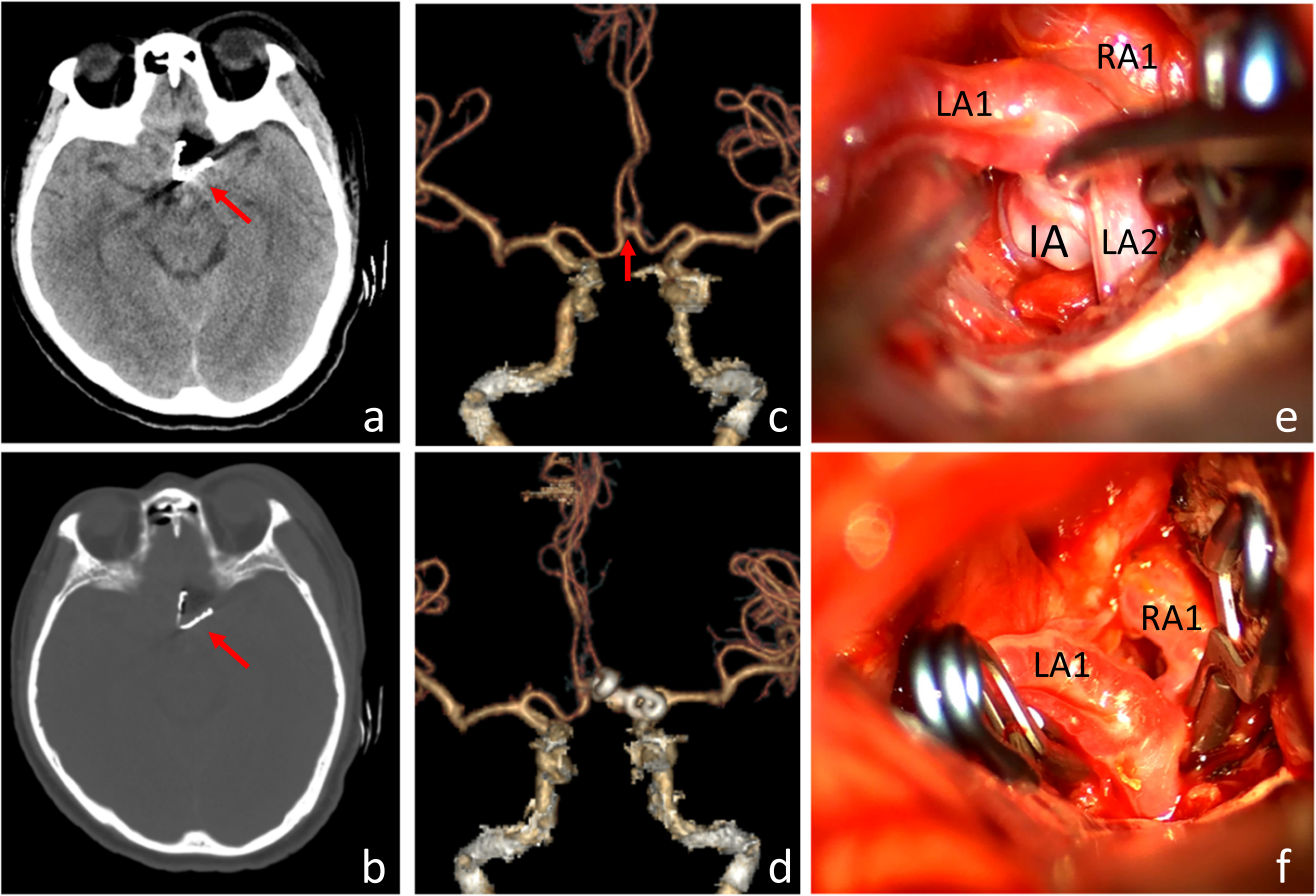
Preoperative and postoperative imaging and intraoperative findings of ruptured anterior communicating aneurysms with reconstruction clipping via supraorbital lateral keyhole approach. a, b The ruptured aneurysm of the patient's anterior communicating artery was shaped and clipped with 2 aneurysm clips. The arrow is the aneurysm clip. c, d CTA vascular reconstruction of the head showed that the aneurysm was completely clipped and the branch vessels were preserved. e, f Fully expose the IA neck during operation and clip it. (LA1: A1 segment of left anterior cerebral artery; LA2: A2 segment of left anterior cerebral artery; RA1: A1 segment of right anterior cerebral artery; IA: intracranial aneurysm)
